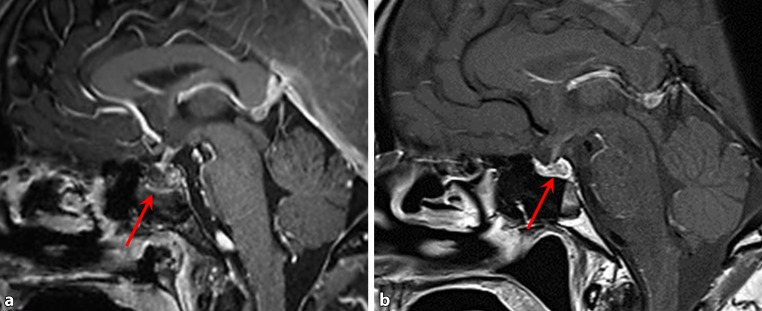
MRI of the brain in the sagittal plane showing reduced contrast enhancement of the enlarged pituitary gland with inhomogeneous contrast enhancement of the pituitary infundibulum (a, *red arrow*) and a reduced volume of the pituitary gland with persistent inhomogeneous enhancement after two months (b, *red arrow*)
